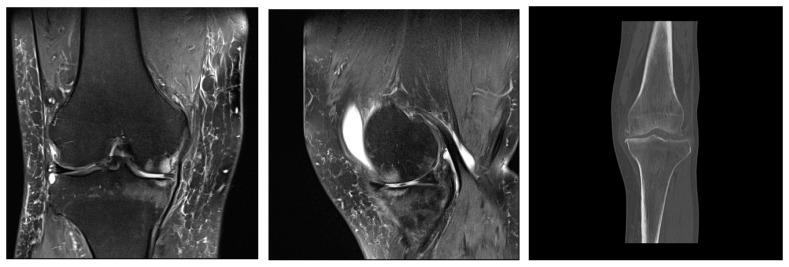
Mild deforming gonarthrosis visualized by MRI (proton-density fat saturated sequences in sagittal and coronal plane) and by CT (multiplanar reconstruction, coronal plane). Patchy areas of subchondral bone edema of femoral and tibial articulations with numerous marginal osteophytes. Articular cartilage is denuded in medial compartment, while in lateral compartment I-II degree hondromalacic changes are seen. Narrowed articular spaces with reactive effusion propagating to suprapatellar bursa are seen. High signal intensity of menisci and cruciate ligaments suggestive of degenerative changes. Ruptured medial meniscus. Reactive edema of periarticular and subcutaneous soft tissues.
